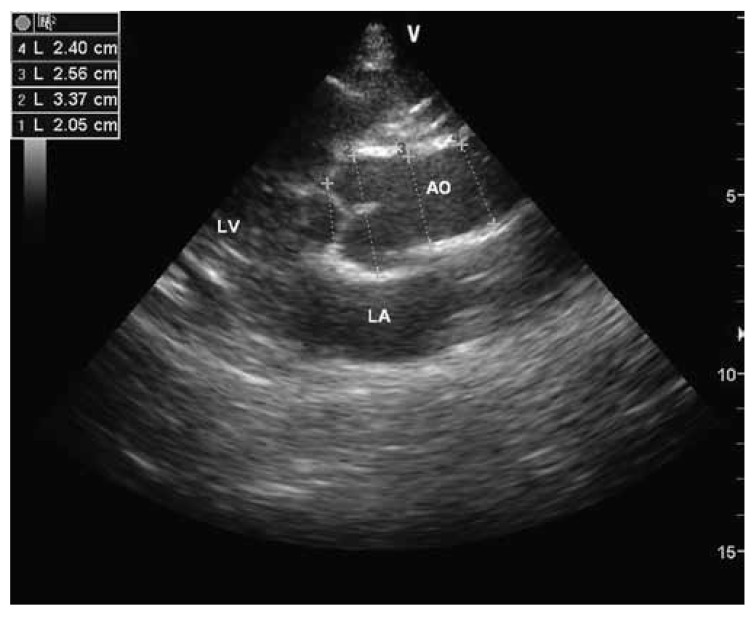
Aortic root measurements with cross-sectional echocardiographic examination. (1) annulus of the aorta, (2) sinuses of Valsalva, (3) supra-aortic ridge, (4) proximal ascending aorta. AO: aorta, LA: left atrium, LV : left ventricle.
